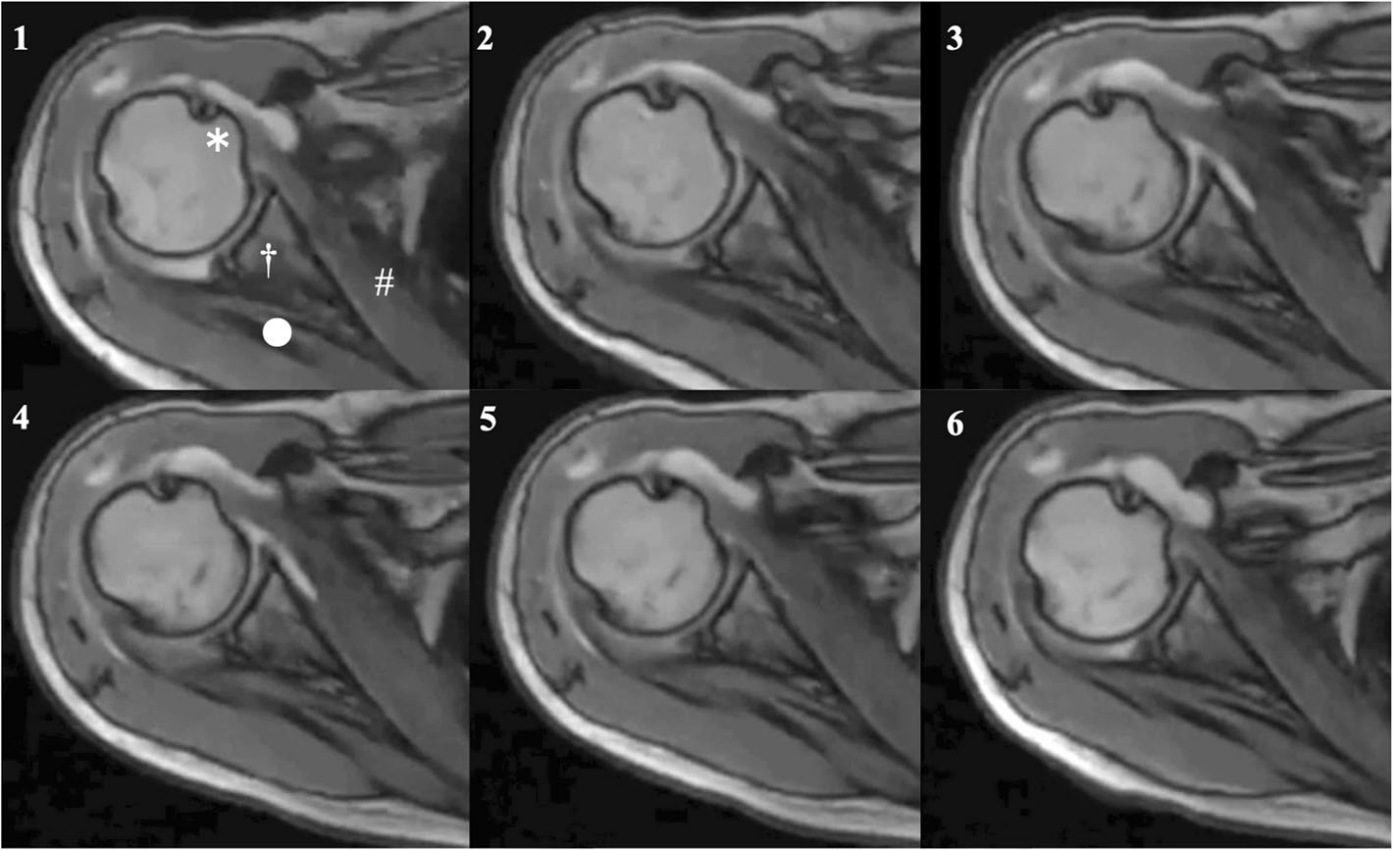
Limitation in external rotation of shoulder with shoulder impingement syndrome. The right shoulder of a patient with shoulder impingement syndrome. Cine-MRI also produced clear images of the movements of the glenohumeral joint rotation in a shoulder with SIS. 1, Starting position; 2, Motion in external rotation; 3, Point at the maximum external rotation; 4 and 5, Motion in internal rotation; 6, End position. *, The lesser tuberosity of the humeral head; #, The subscapularis; †, Glenoid; ○, The infraspinatus
